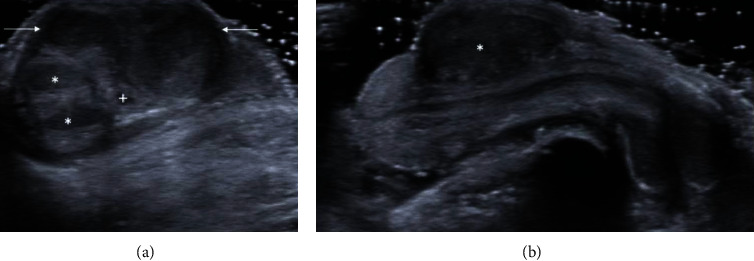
Grey scale ultrasound of the penile median raphe cyst. (a) Transverse view of the distal penile shaft demonstrating the heterogeneous solid mass (arrows) in relation to the corpora cavernosa (∗) and corpus spongiosum (+). (b) Sagittal view demonstrating the solid-appearing median raphe cyst (∗) at the ventrolateral aspect of the distal penile shaft.
